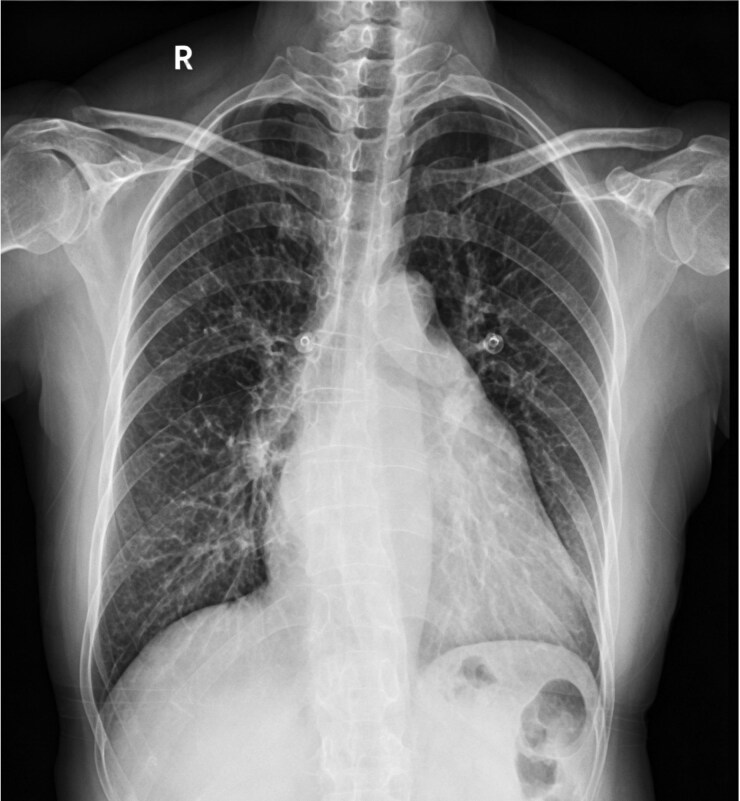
Preoperative chest radiographs.
